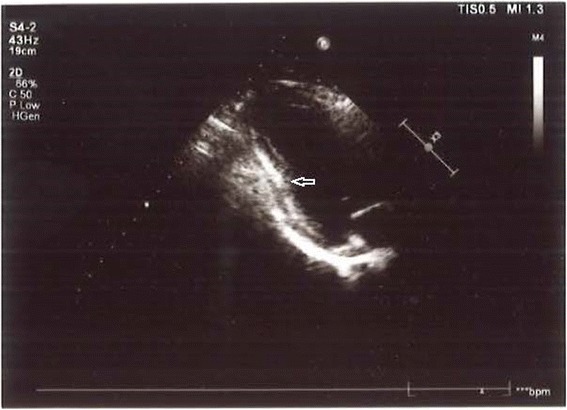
Focal echobright sign in posterolateral wall in echocardiography in acute myopericarditis (Patient 1)
